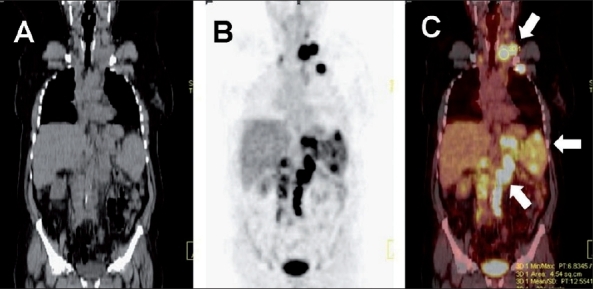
Disseminated TB with focal splenic lesions, sparing of the liver and with extensive lymph node involvement. Coronal CT (A), PET (B), and PET/CT (C) images in a patient with cervical lymphadenopathy show large FDG-avid cervical, supraclavicular, and abdominal nodes. FDG-avid focal lesions are seen in the spleen (arrows). The differential diagnoses included lymphoreticular malignancy (lymphoma), metastases from an unknown primary, and tuberculosis. A supraclavicular lymph node biopsy confi rmed tuberculosis. Note the relative sparing of the liver
